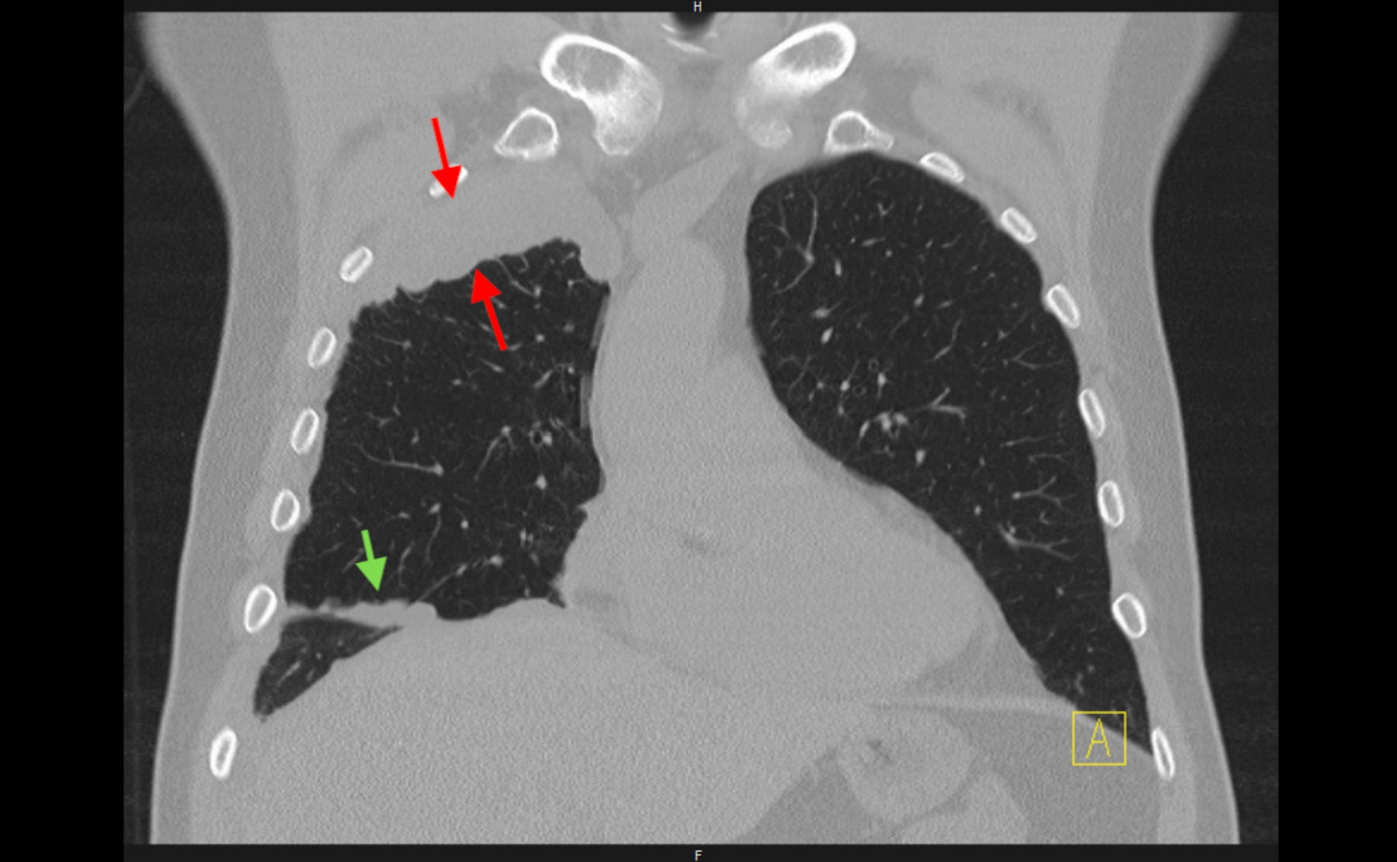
Coronal cross-section of CT Chest. Right upper lobe pleural mass (red arrows) with nodularity of the right oblique fissure (green arrow).
